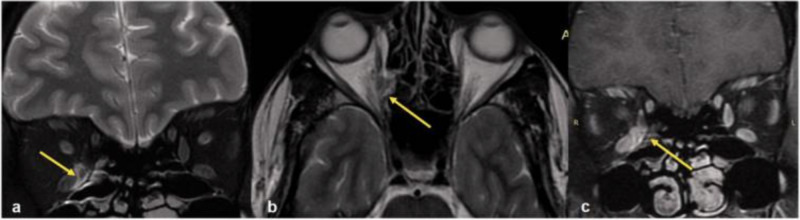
MRI of orbit Coronal (a), axial (b) T2W MR image shows a hyperintense lesion medial to the medial rectus muscle (yellow arrow) extending to the medial orbital wall forming sub-periosteal abscess and extending laterally into the intraconal fat. Post-contrast coronal T1W image (c) shows the enhancement (yellow arrow). This was a case of orbital cellulitis. T2W: T2-weighted; T1W: T1-weighted This image was obtained with consent from The Neuroradiology Journal [3].
